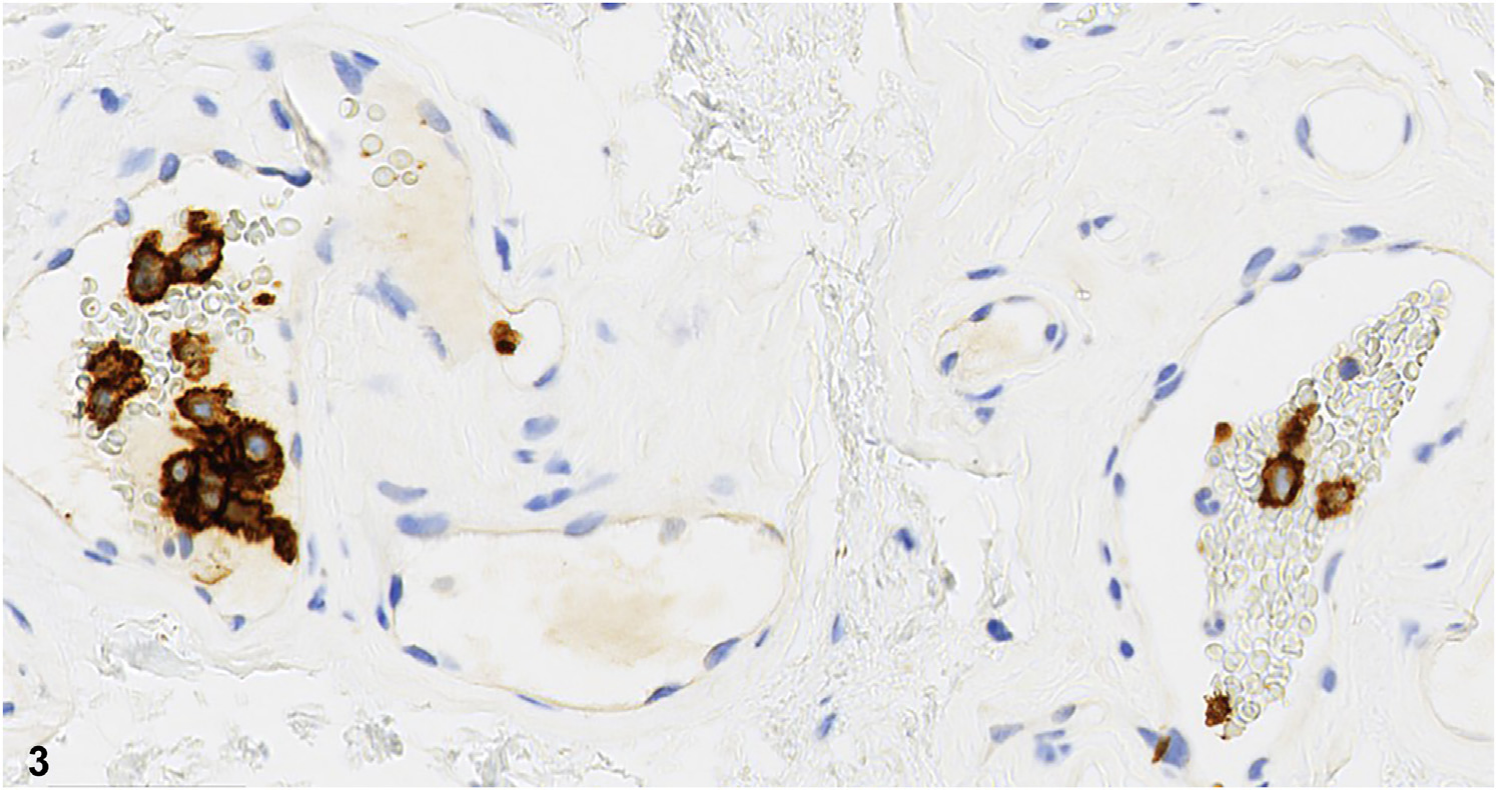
Magnetic resonance imaging (MRI) revealed punctate frontal lobe infarcts and hyperintense lower thoracic cord signal with cauda equina involvement. Lumbar puncture showed elevated protein with lymphocyte pleocytosis, and laboratory tests revealed no evidence of lymphoproliferative disorder.
